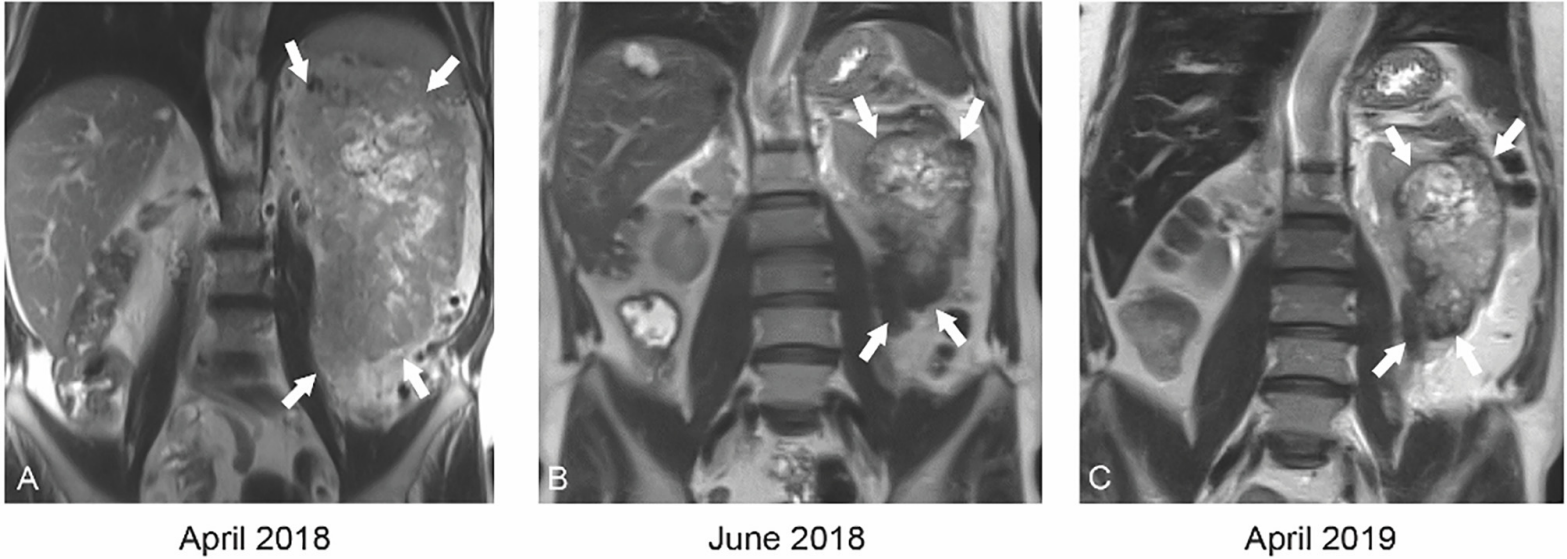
Coronal T2 weighted MRI at baseline (A) demonstrates a large 21.7 cm mass (white arrows) replacing the entire left kidney with central areas of necrosis. After just 2 months of cabozantinib therapy (B), the mass had decreased to 12.1 cm (white arrows). After 12 months of cabozantinib (C), the mass was stable in size.
